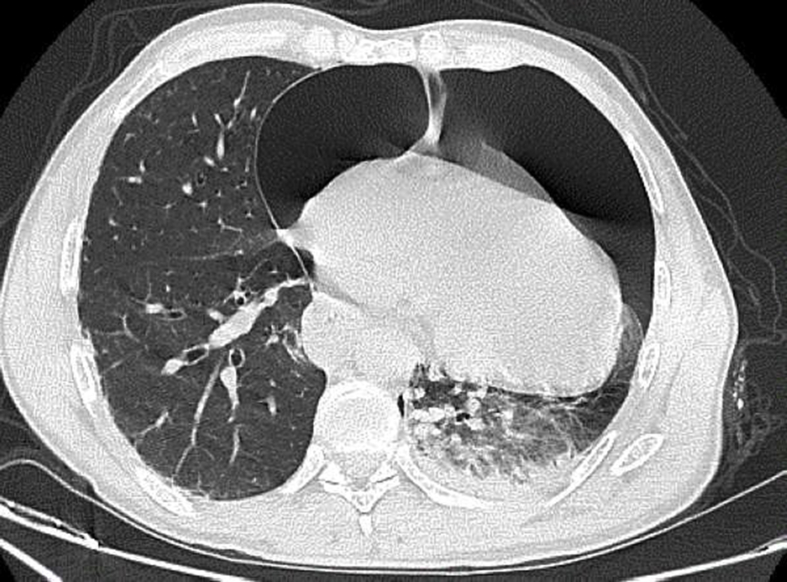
Computed tomography scan on admission, axial plane. An expressive non-tension pneumopericardium communicating with a large left pneumothorax. Left lung contusion can also be noticed.
